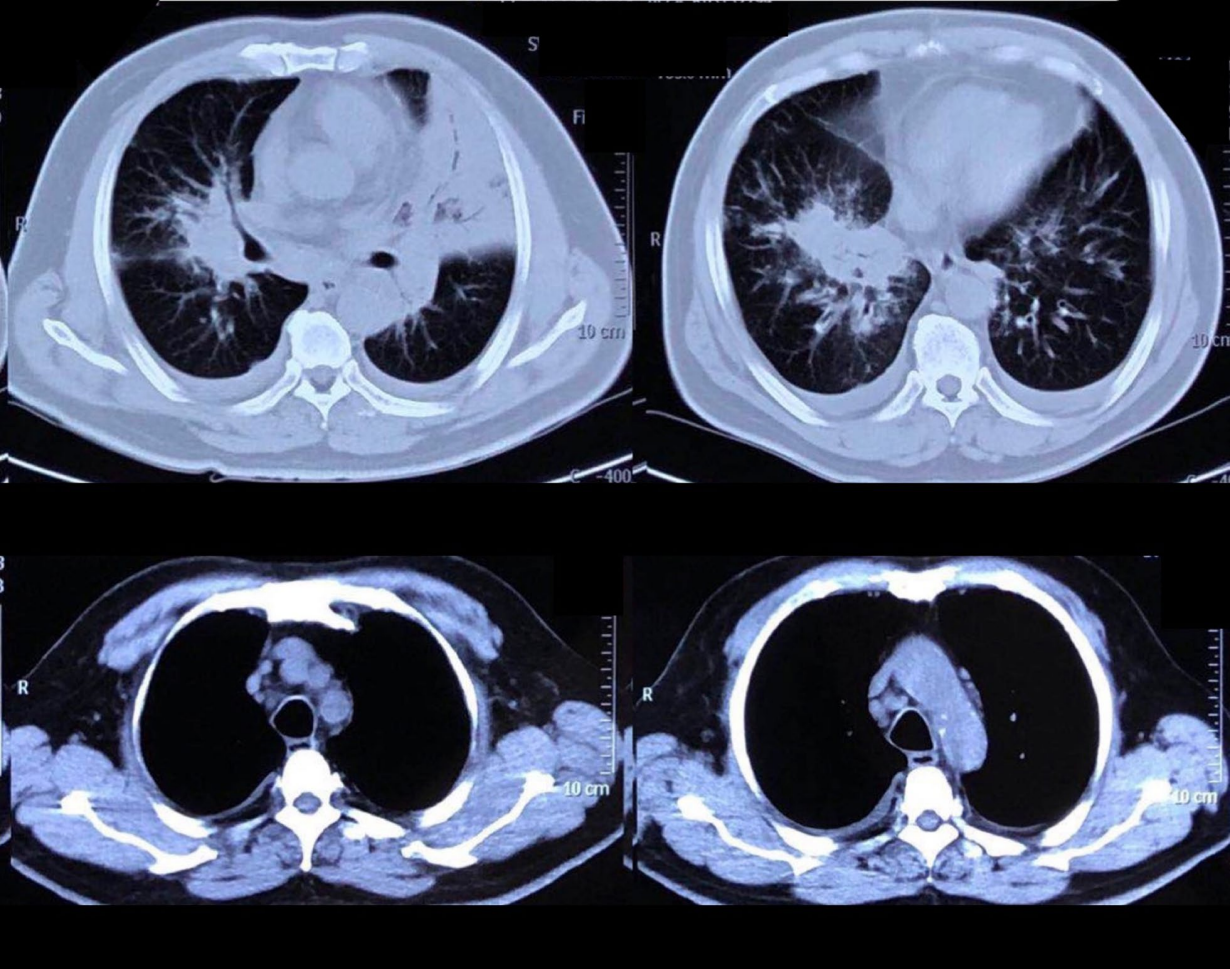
CT scan on admission: multiple patchy opacities with consolidation in both lungs, marked lesions in the left lung, and multiple lymphadenopathy in the mediastinum and hilum
